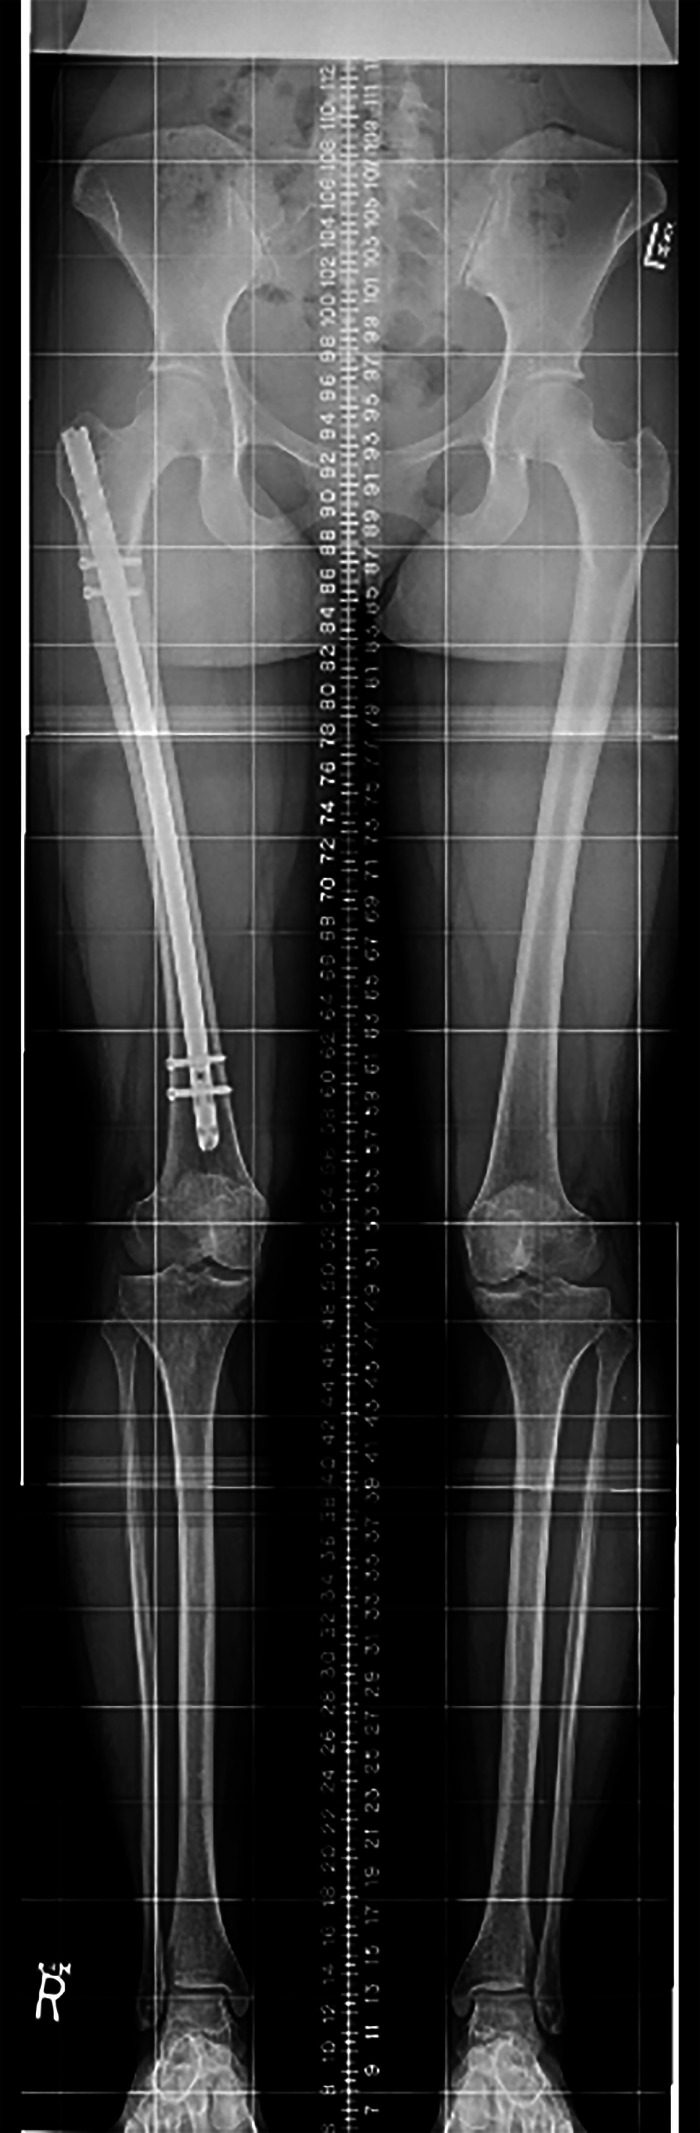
Hip to ankle long leg standing radiograph shows healed right femoral osteotomy with 2° valgus and left knee neutral alignment.
